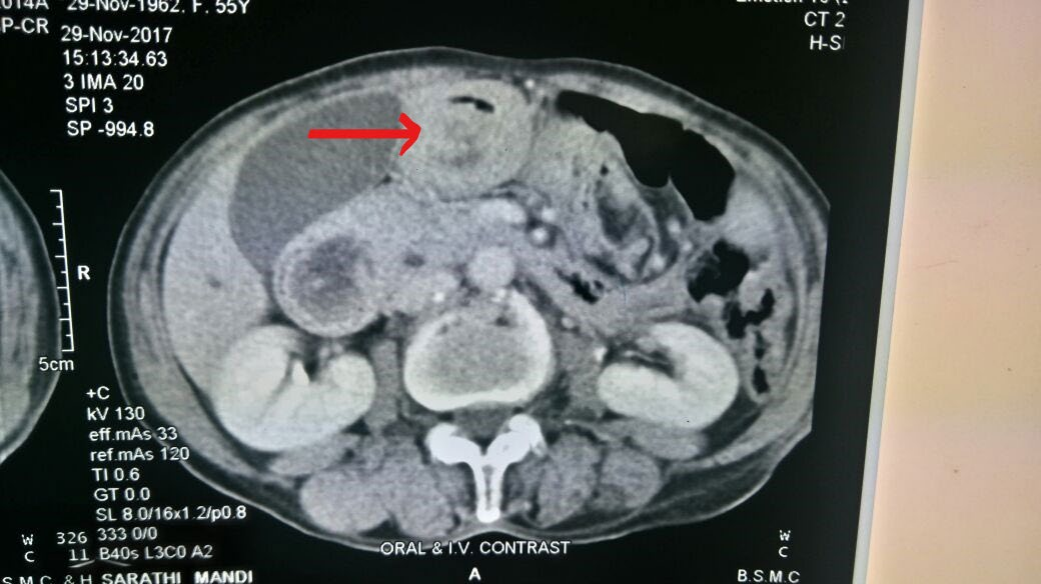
CT scan showing gastric tumor invaginating into second part of duodenum (red arrowhead)
